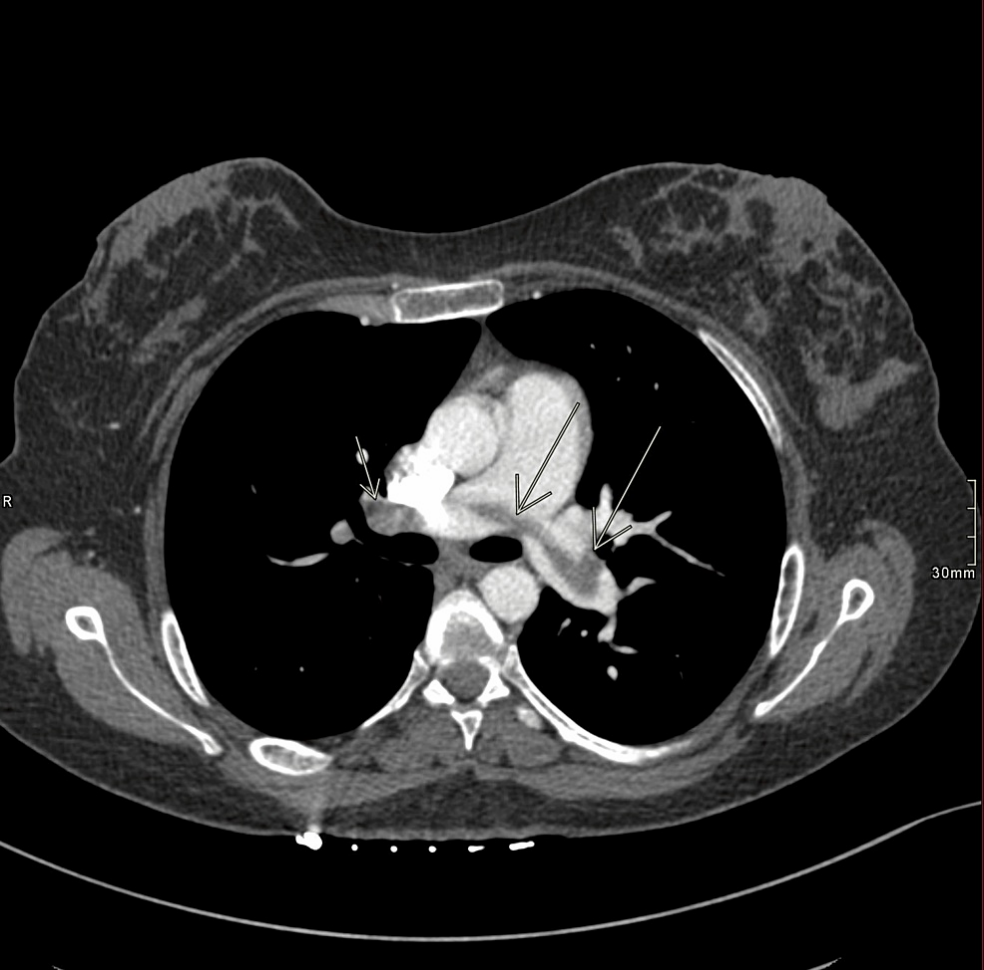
Transverse CT pulmonary angiogram demonstrating the largest transection of the pulmonary thrombus, which measured approximately 0.5 cm wide and spanned over 10 cm from end to end in the main pulmonary arteries before branching into subsegmental arteries (not included in this view).
